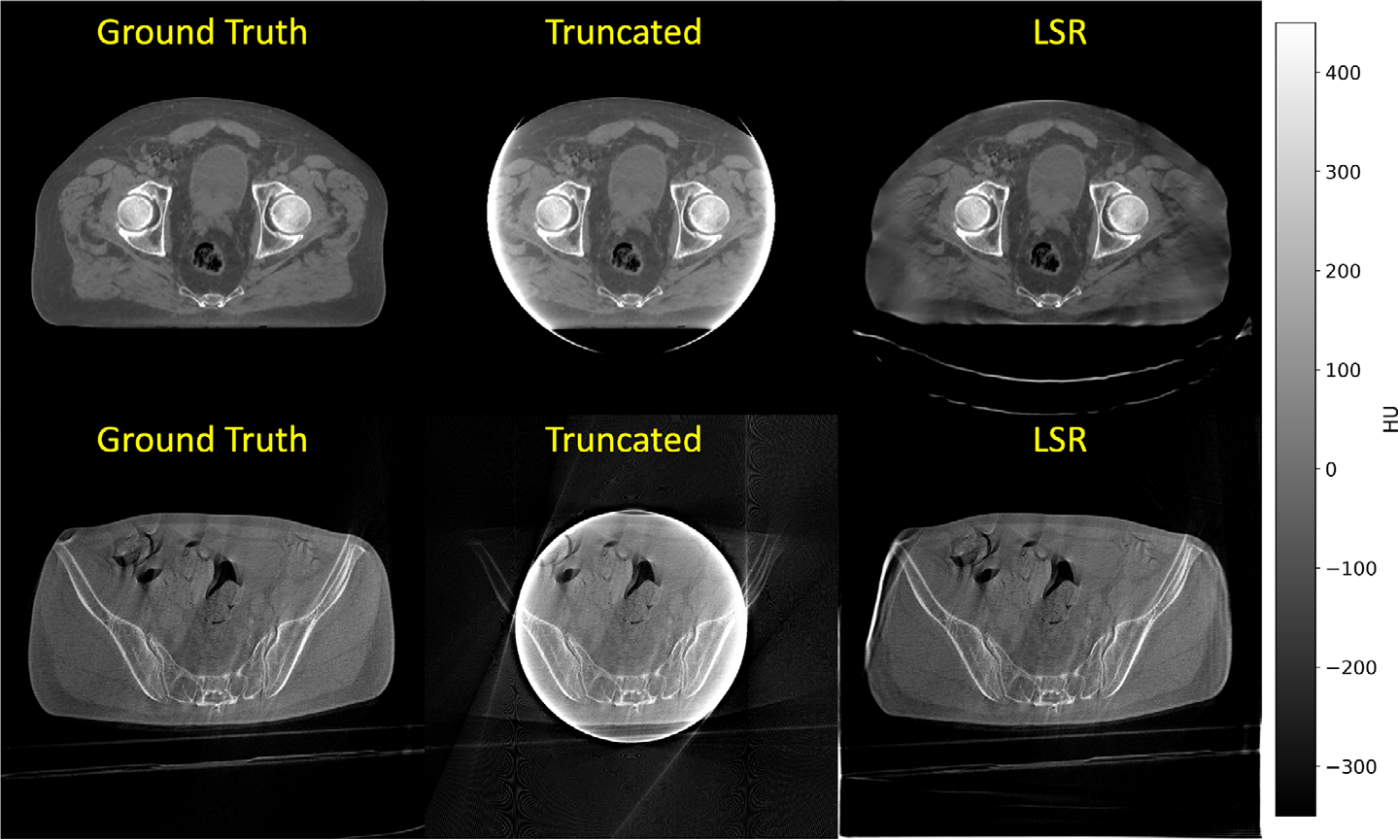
Detruncation of two CBCT measurements at a 20 cm FOV. Top row: Measurement from an onboard CBCT of a Varian ProBeam360 with a tube voltage of 140 kV. Bottom row: Measurement from HyperSight with a tube voltage of 125 kV. Window level: C=50 HU, W=800 HU.
